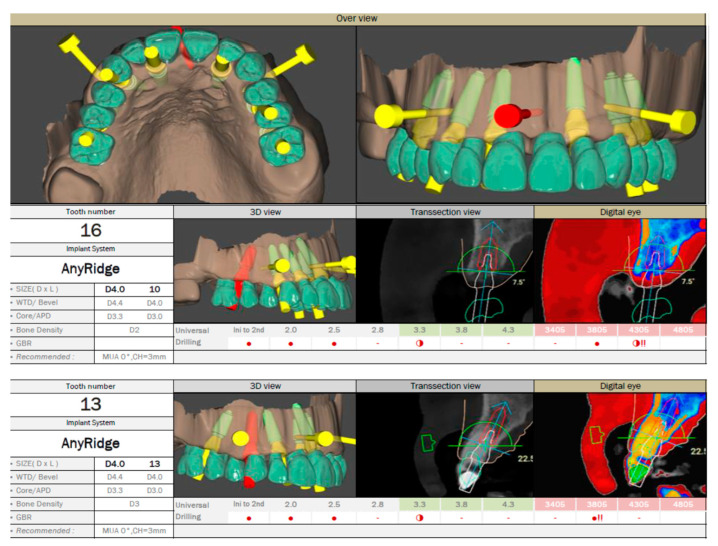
(Patient #2): Detailed positioning of the implants and transversal pins generated by R2Gate™ software according to the surgical plan. Bone density and the recommended drilling sequence for each implant position are also displayed. The R2Gate™ software converts the CBCT grayscale into five basic colors corresponding to the 256 shades of gray (right images): black represents air, red indicates soft tissue, blue represents soft bone, yellow corresponds to dense bone, and green represents high-density structures (such as enamel, cortical bone, and metal structures).
